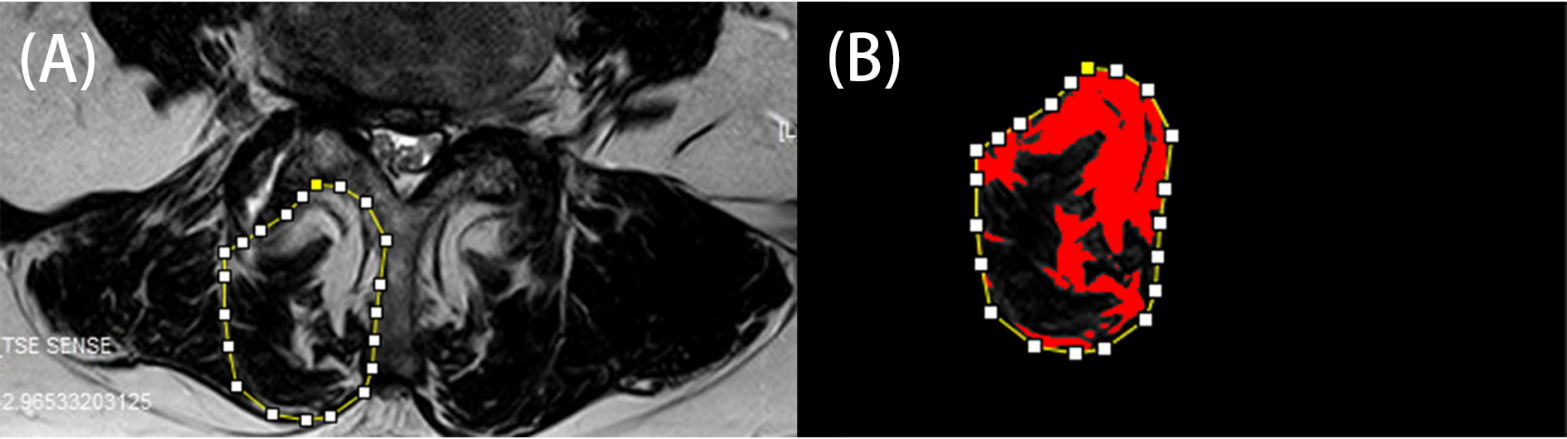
LMM similar in shape and quality. (A) Preoperative MRI examination. (B) Postoperative MRI examination.
